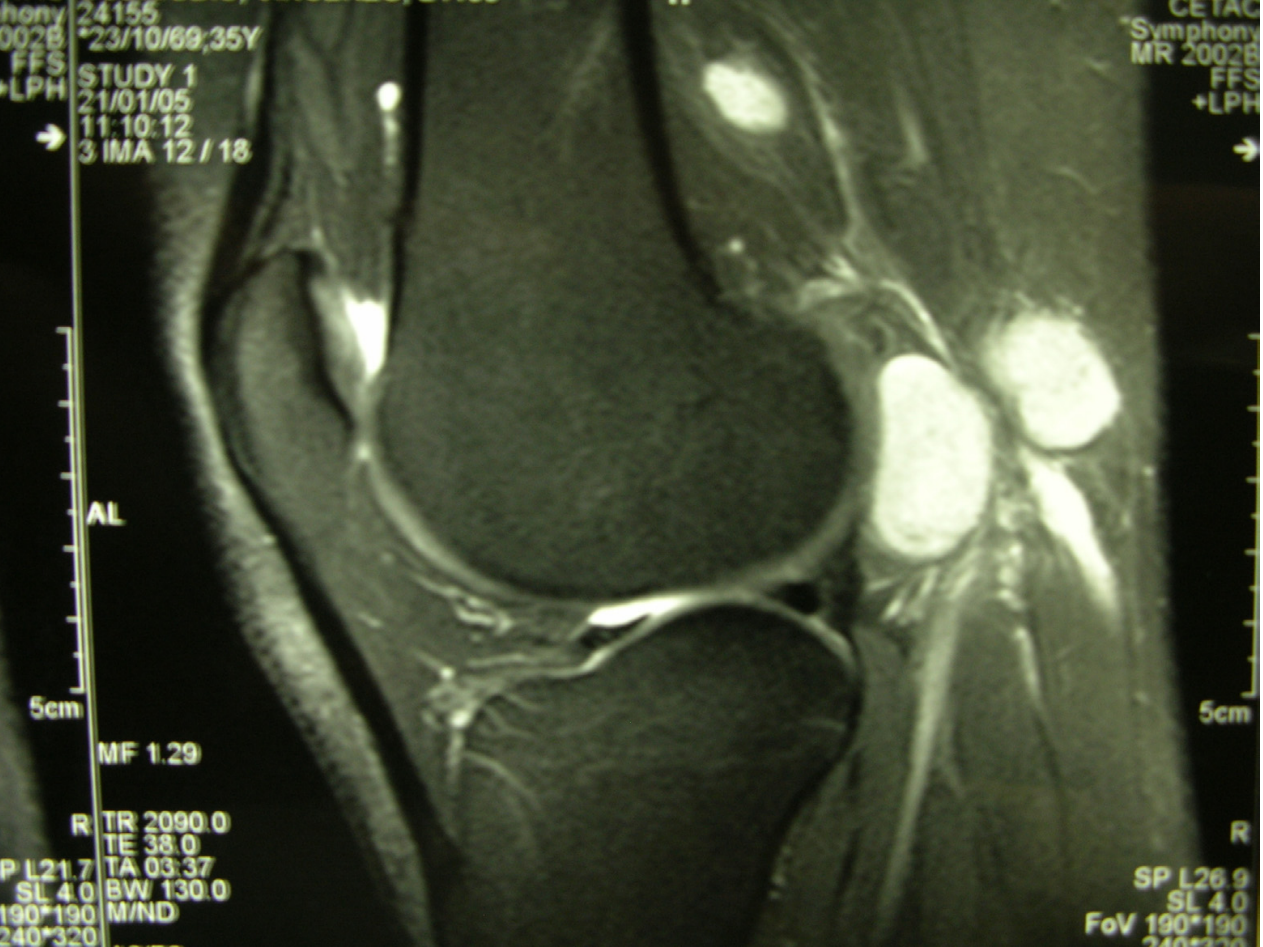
Sagittal MRI of the left popliteal fossa showing two involved nodes anterior to the neurovascular bundle.
